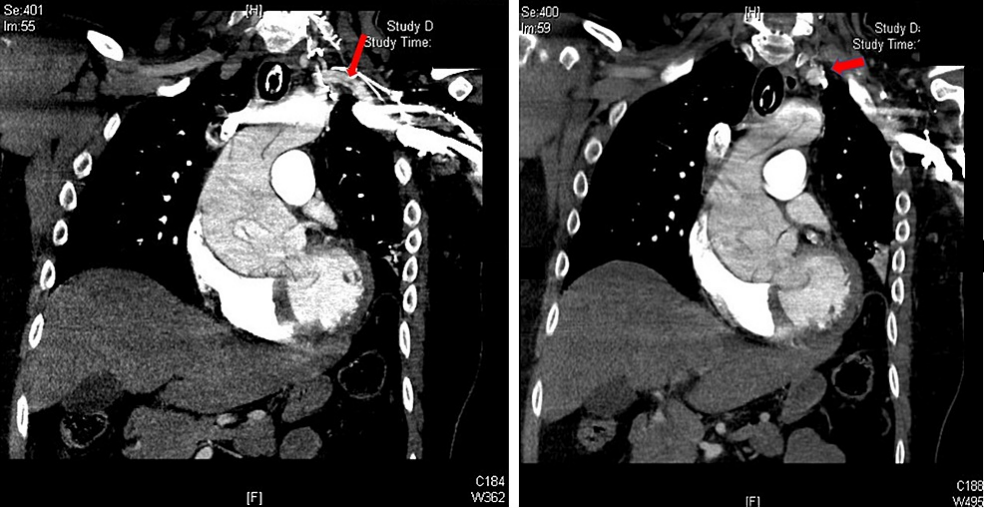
CT angiogram demonstrating Stanford type A aortic dissection (arrows) H: head, F: foot.
